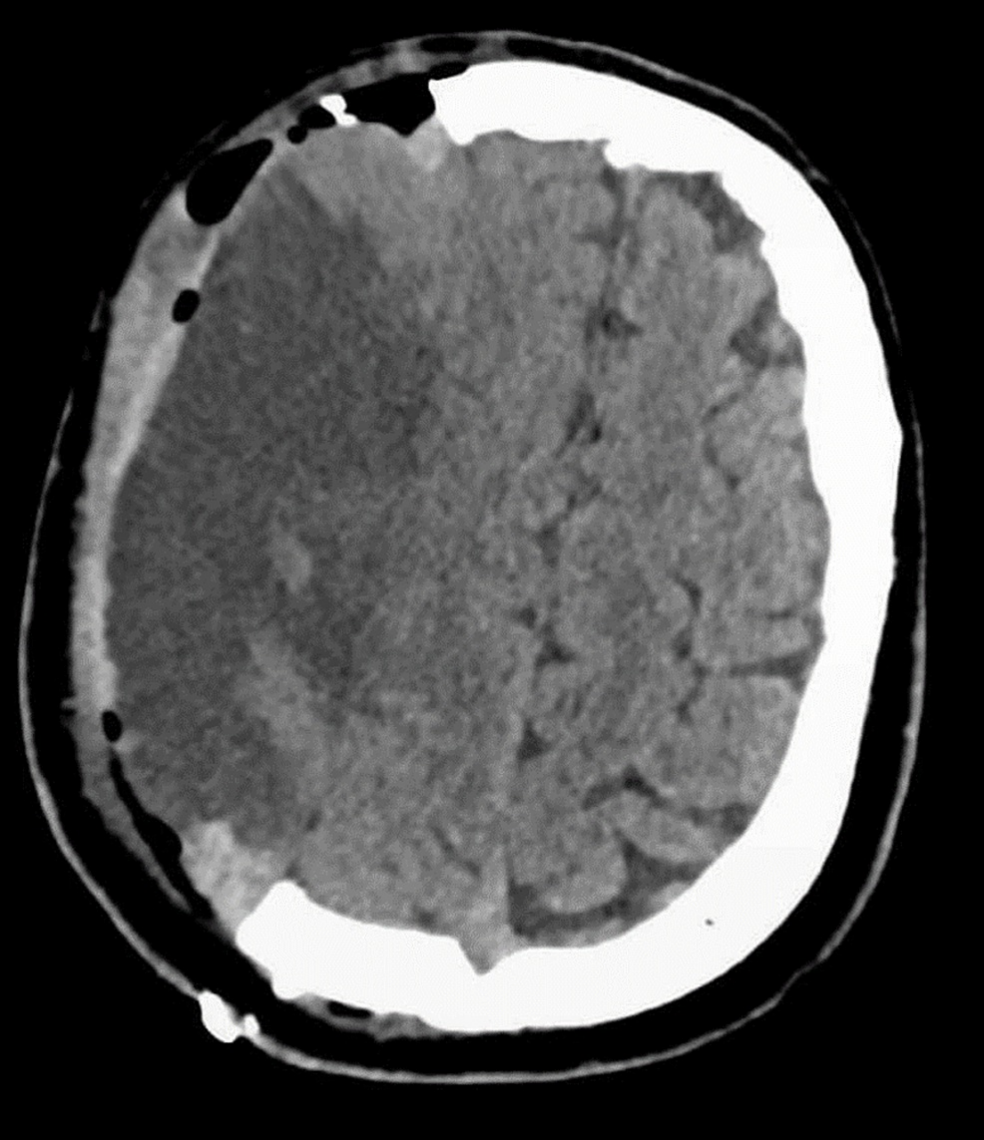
Non-contrast CT head showing postoperative changes at the right hemicranium in the form of right frontoparietal craniectomy and extra-axial hematoma CT: Computed Tomography
